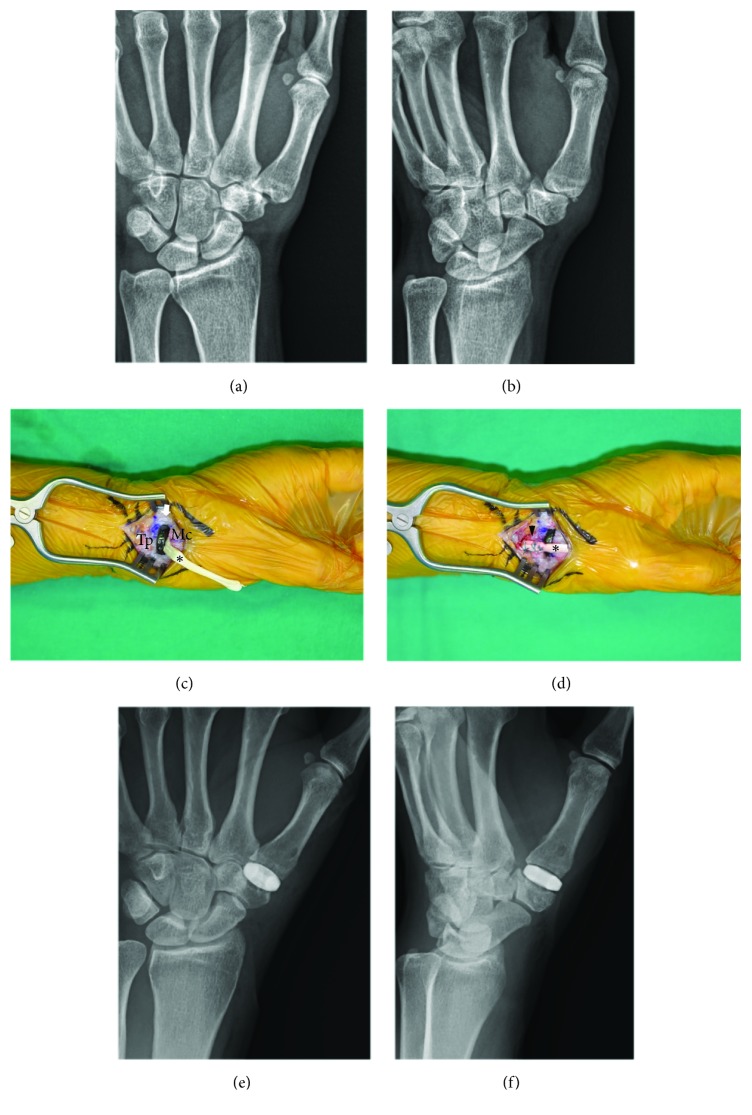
The pyrocarbon interposition arthroplasty surgical procedure. (a, b) Posteroanterior and 45-degree oblique radiographs of a 62-year-old female with Eaton-Littler stage II trapeziometacarpal osteoarthritis. (c) The PyroDisk implant (arrow) was inserted in the trapezium space after resecting both the base of the first metacarpal bone and the distal trapezium using a sagittal saw and 3-mm burr to create concavity. The half flexor carpi radialis (FCR) tendon (*∗*) was passed through the trapezium into the resected joint through the selected PyroDisk implant. (d) The FCR tendon then was continuously passed into the thumb metacarpal bases to exit dorsally through the prepared passage and sutured to the inserted portion of FCR tendon (arrowhead). (e, f) Posteroanterior and 45-degree oblique postoperative radiographs. Mc, metacarpal bone; Tp, trapezium.
